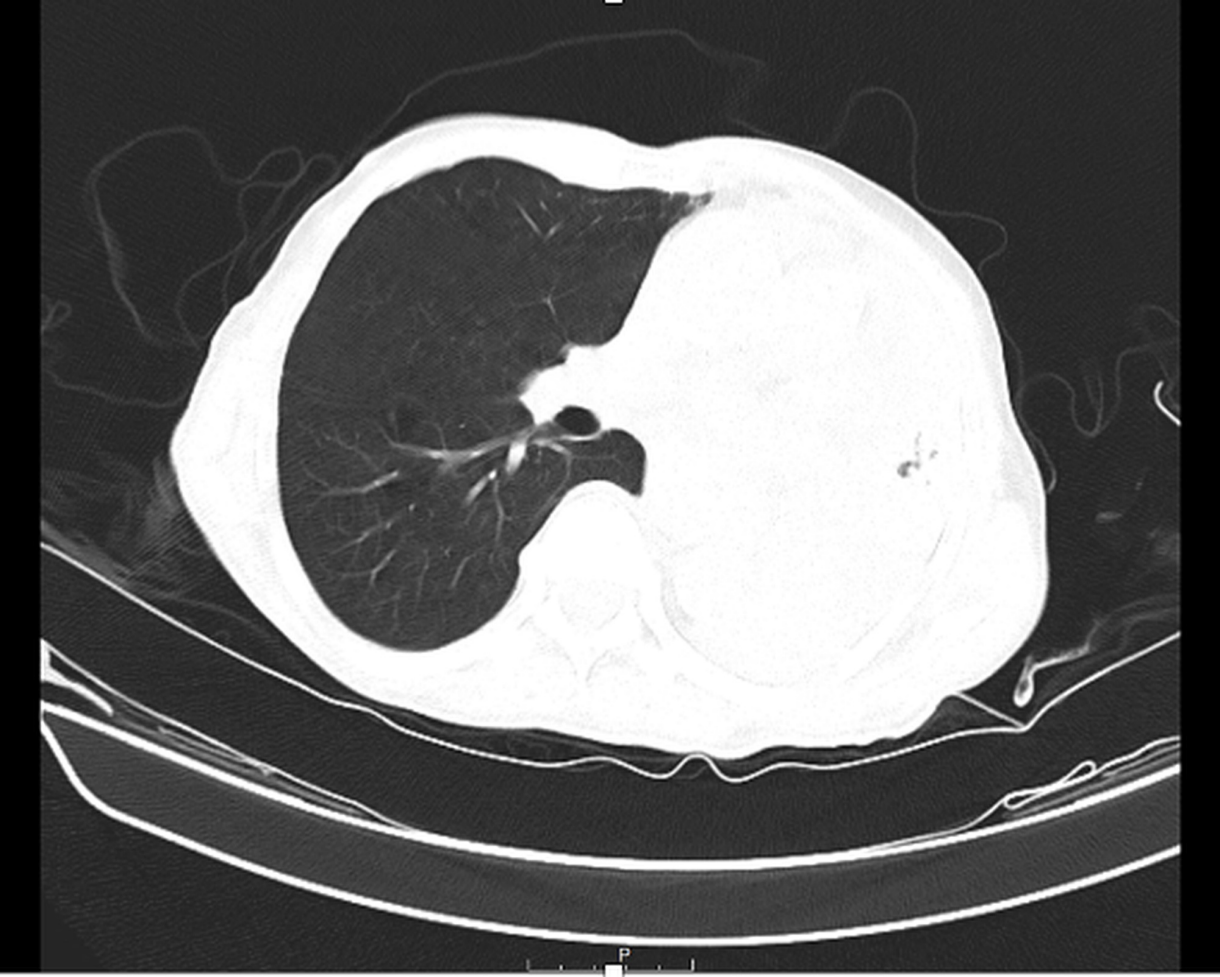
CT chest revealed retained barium within the upper to the mid-thoracic esophagus. Complete left lung atelectasis was noted, likely related to the obstructing filling defect within the left main bronchus.
